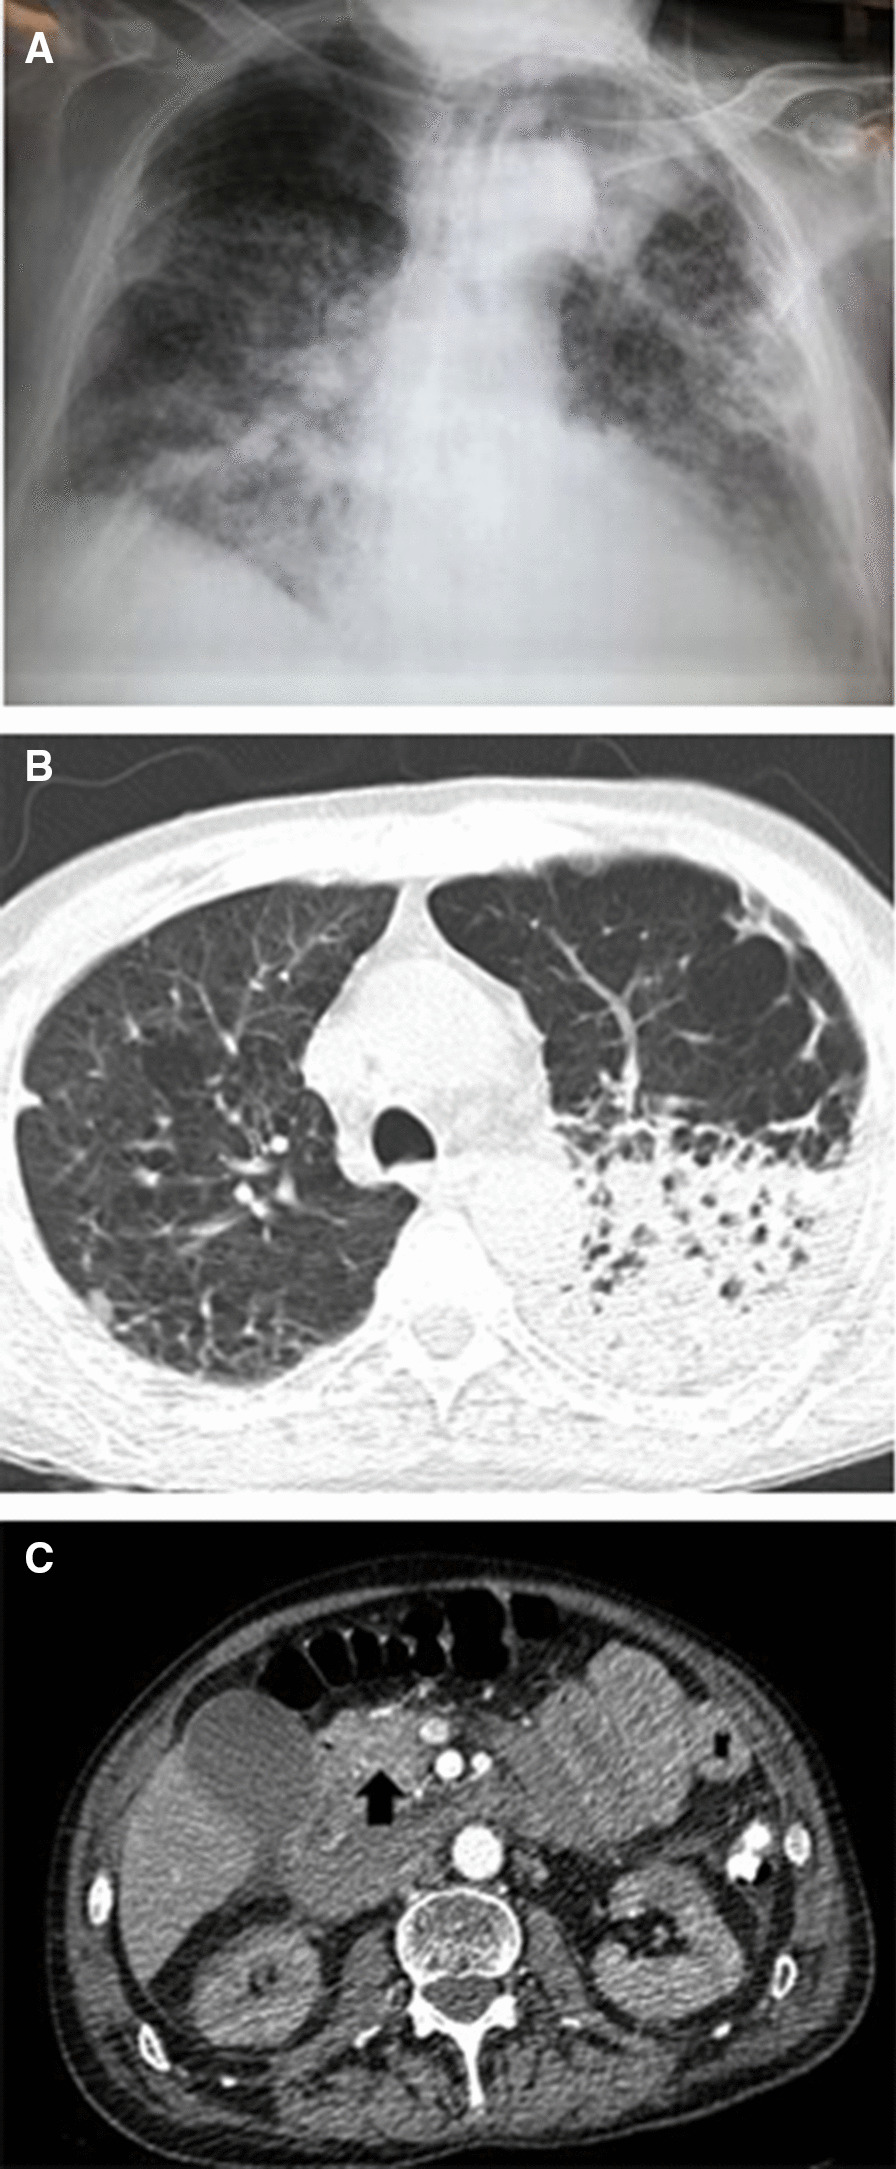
a CXR of the patient on admission showing infiltrative shadows in both lungs, especially in the left upper lobe. b Chest CT scan showing multiple intraparenchymal cavitated nodules in the left lung. c Abdominal CT scan showing a large retroperitoneal hematoma (arrow) and multiple aneurysms of the renal, hepatic, and pancreaticoduodenal arteries
